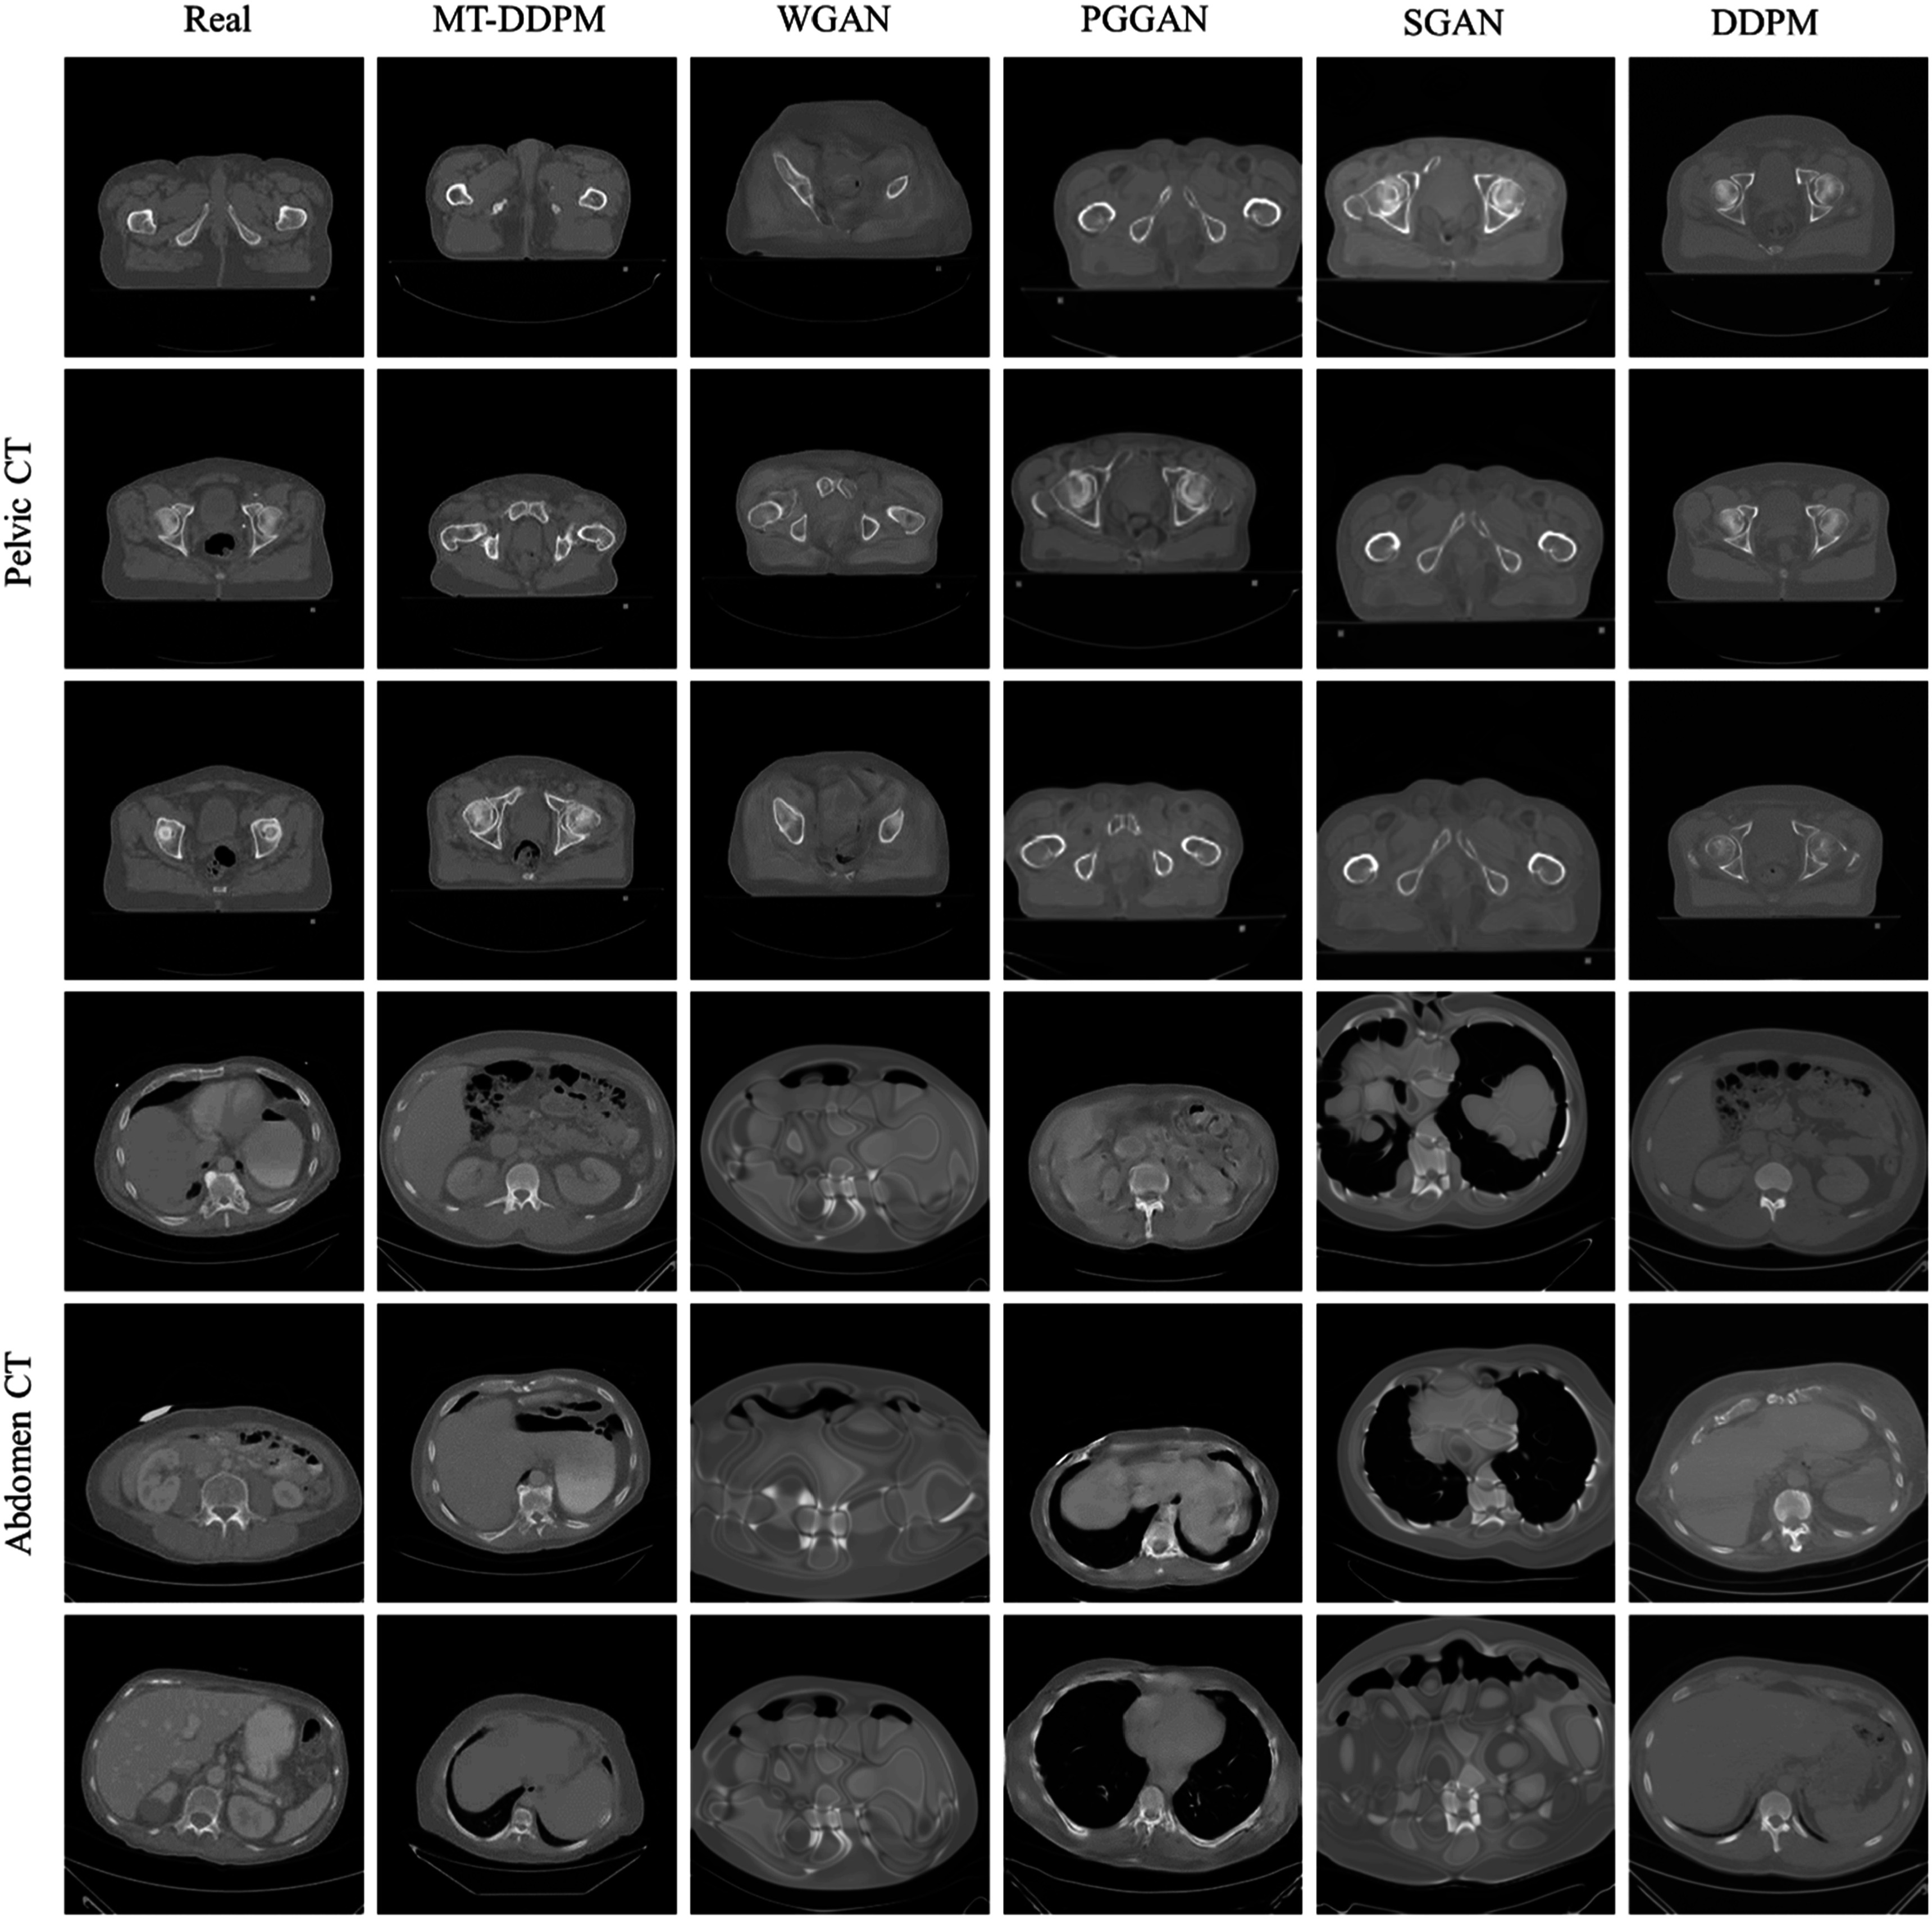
Synthetic and real images from pelvic and abdomen CT. In each dataset, the real images, the real images, the synthetic images of the proposed MT-DDPM, WGAN, PGGAN, SGAN, and DDPM are presented in column-wise. Three slices of pelvic CT images (row #1 to #3), and abdomen CT images (row #4 to #6) are displayed.
